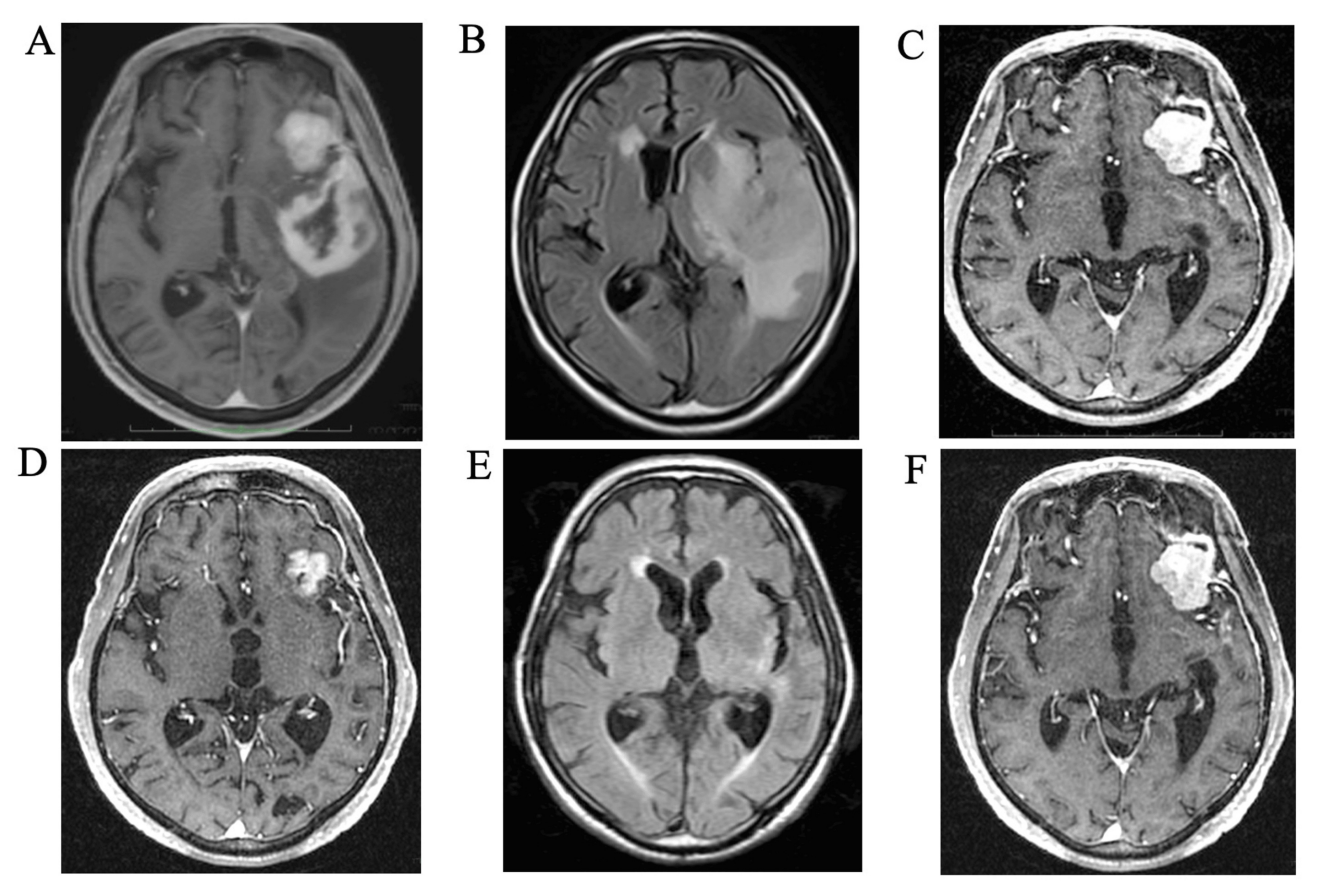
MRI with Gd enhancement (T1-Gd) (A) Initial T1-weighted MRI with Gd enhancement (T1-Gd) shows a large intra-axial tumor with ring enhancement. (B) FLAIR MRI shows the intra-axial tumor causing brain edema around the tumor. (C) T1-Gd shows a large extra-axial tumor attached to the dura with homogeneous enhancement. (D) T1-Gd five months after Bev administration shows disappearance of enhanced lesion of the intra-axial tumor and a slight reduction in the size of the extra-axial tumor. (E) FLAIR MRI shows disappearance of expanding perifocal edema surrounding the tumor. (F) Compared with (C), T1-Gd five months after Bev administration shows no remarkable differences in the volume of the extra-axial tumor. MRI: magnetic resonance imaging, Gd: gadolinium, FLAIR: fluid-attenuated inversion recovery, Bev: bevacizumab
